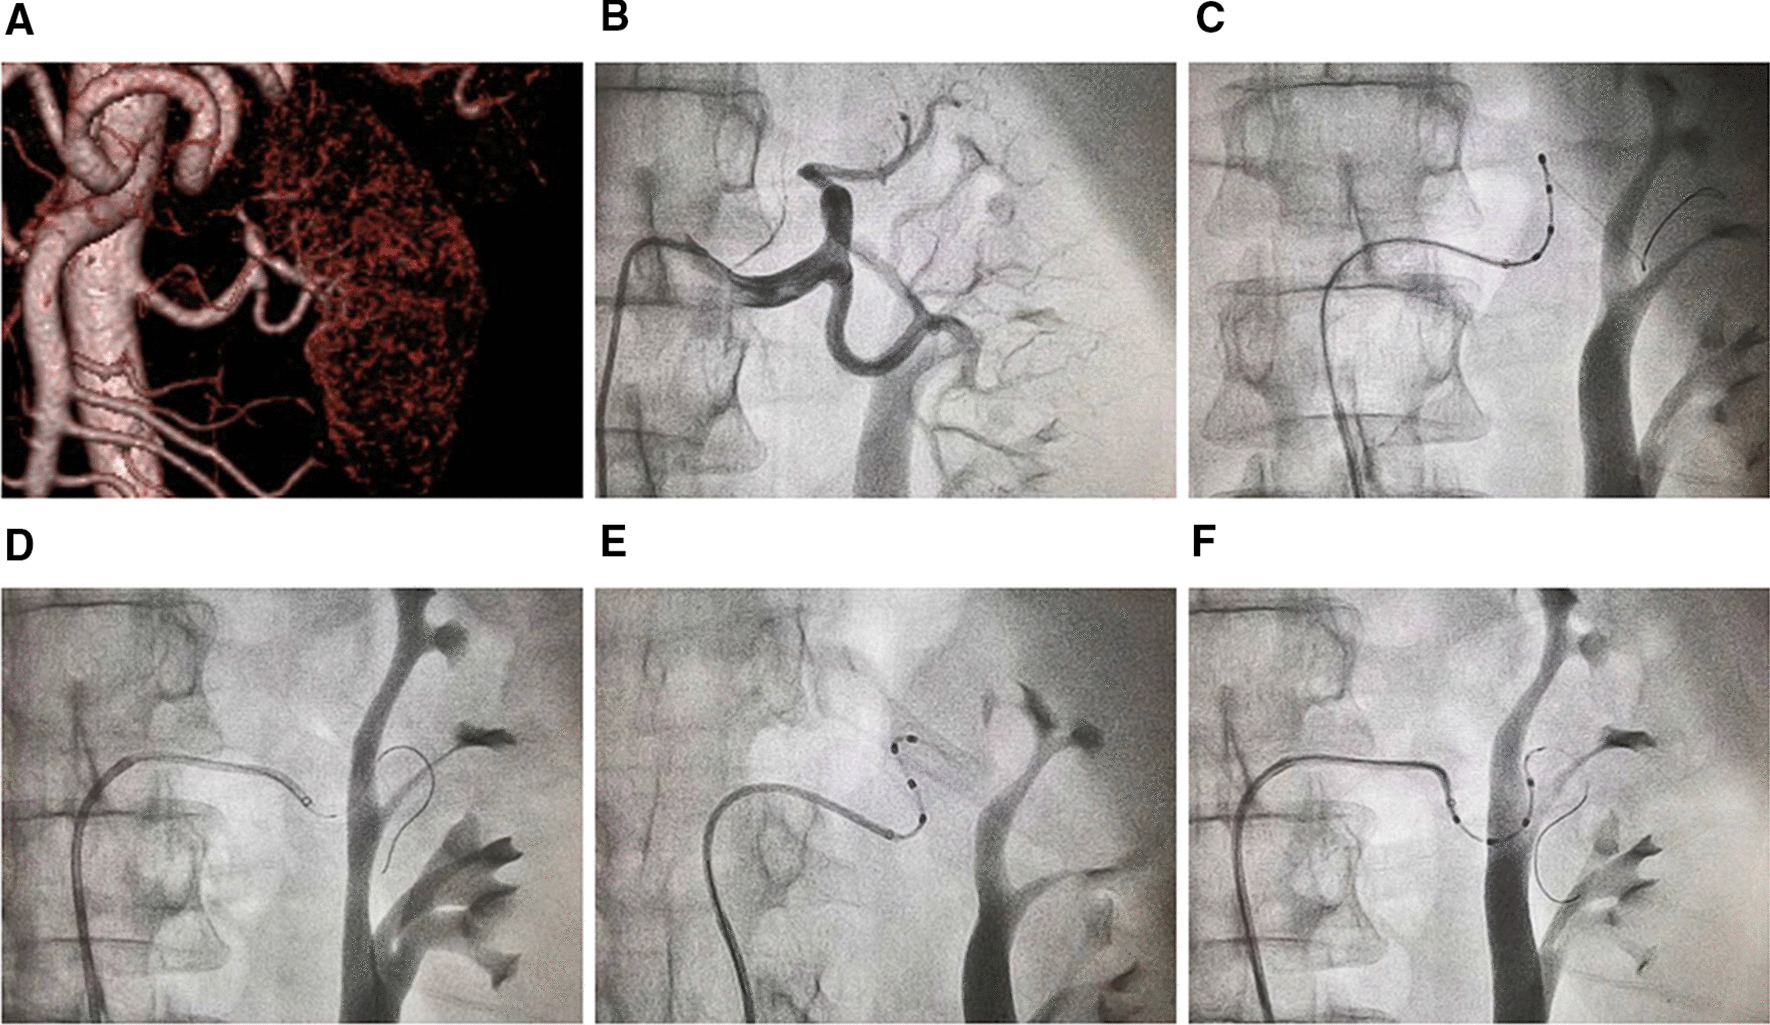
Angiography and denervation of the left renal artery. a Three-dimensional reconstruction of computed tomography angiography showing the highly tortuous left renal artery. b Angiography of the left renal artery. c Attempted ablation using an Iberis ablation catheter with a regular guiding catheter alone. d Insertion of a guide extension catheter. e Denervation of the upper branch of the left renal artery using a guide extension catheter. f Denervation of the lower branch of the left renal artery using a guide extension catheter
